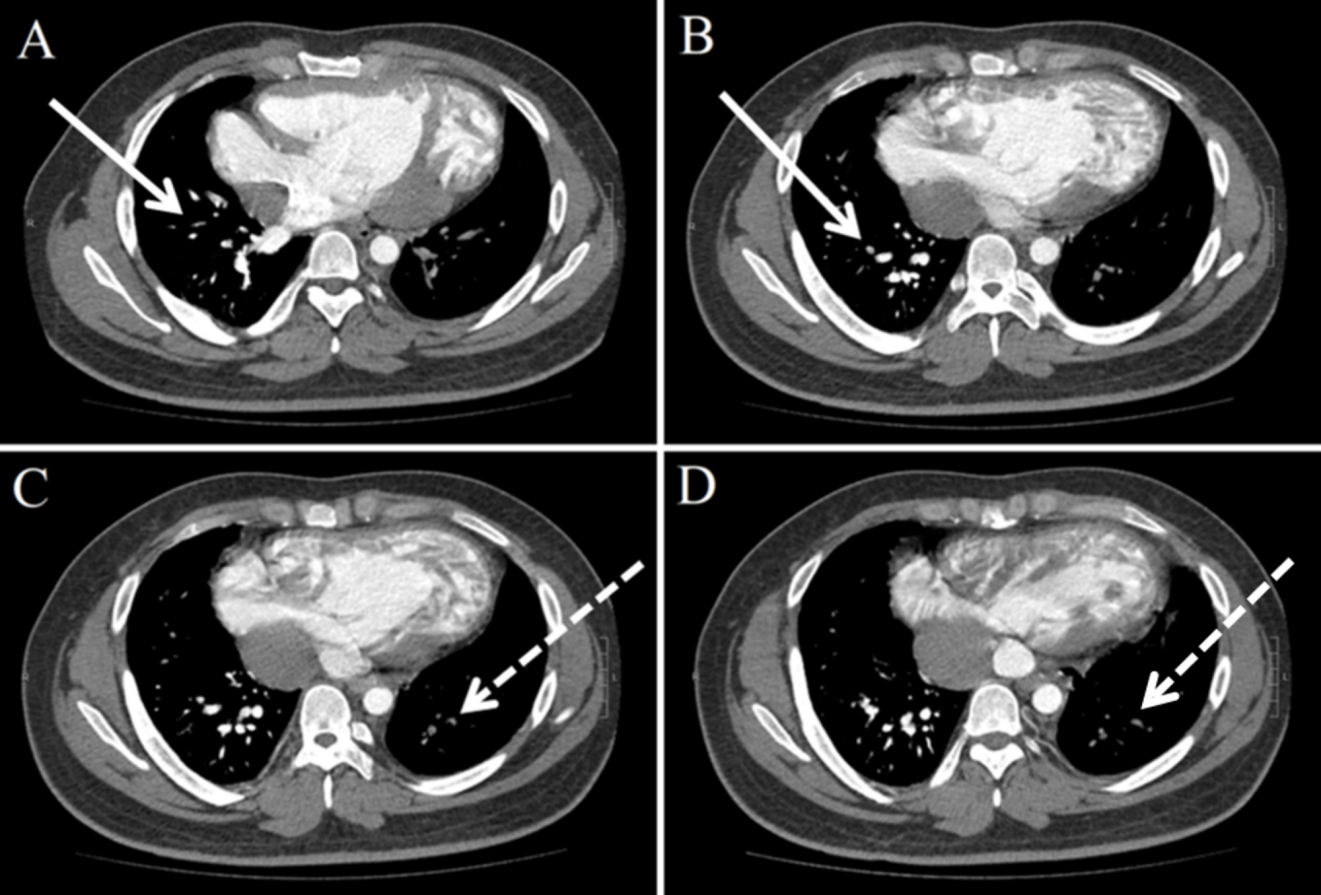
Axial CT pulmonary angiogram demonstrating SVC to be contiguous with the right PA with clear opacification (solid arrow line in panes A and B) and also shows IVC to be contiguous with the left PA, but without any opacification (dotted arrow line in panes C and D). SVC: superior vena cava; PA: pulmonary artery; IVC: inferior vena cava
